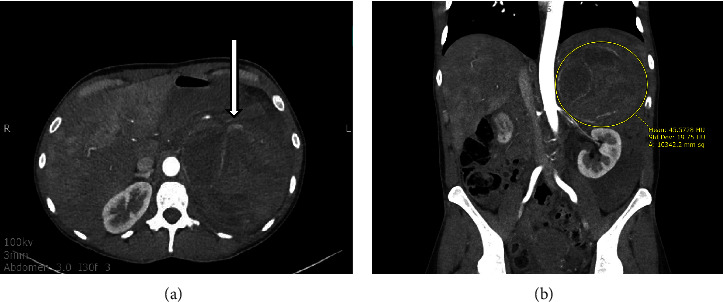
CT abdomen/pelvis with contrast showing a heterogenous 12.5 cm × 10.7 × 9.4 cm left adrenal mass coronal view (a) and axial view (b).
